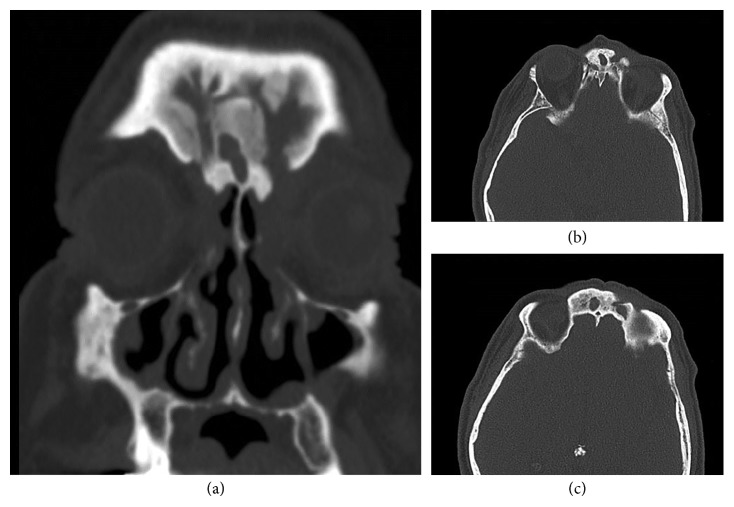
CT orbit, sinuses, and skull base. Small A-P diameter of frontal sinus with extensive neo-osteogenesis secondary to chronic frontal sinusitis.
